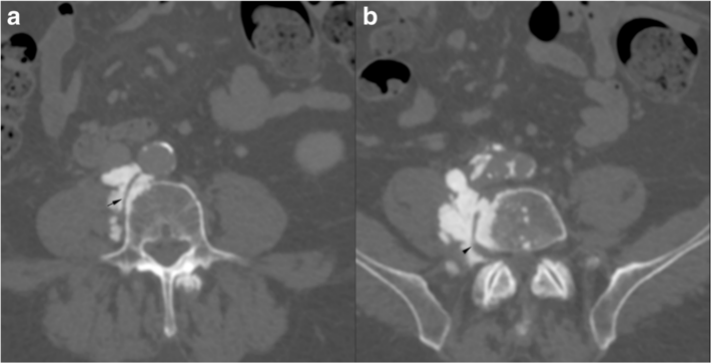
Axial CT slices (a, b) depict the sheating of the vessels by the ossified mass (arrow) as well as the sheating of the lumbar nerves (arrowheads)
